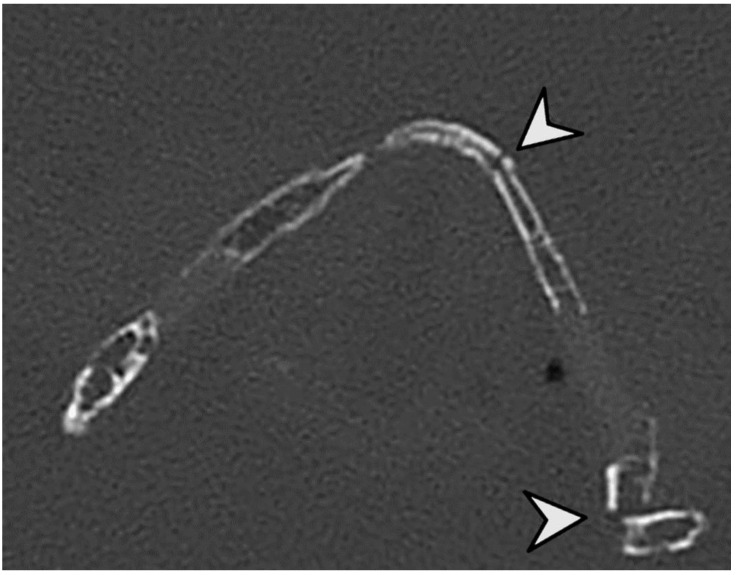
Post-mortem CT of the larynx after reconstruction, where two fractures are visible (in the corpus and the cornu superius). The two fractures are indicated by arrowheads.
